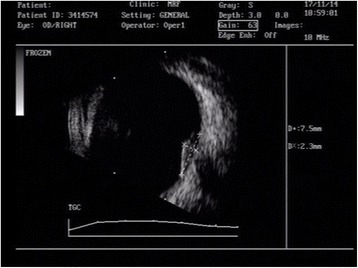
Ultrasound B-scan of the right eye
